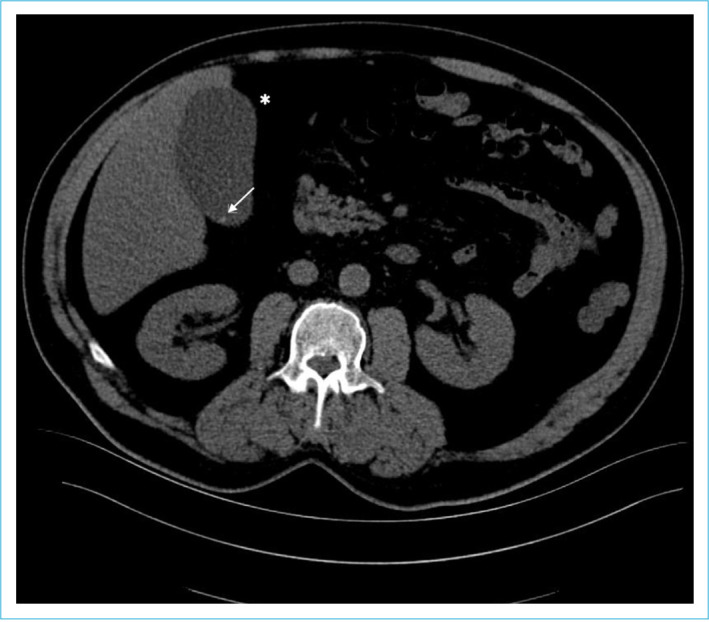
Axial non-contrast CT image shows no abnormal finding related to iron accumulation in kidneys. Note hydropic gallbladder (star) and millimetric gallstone (arrow).
